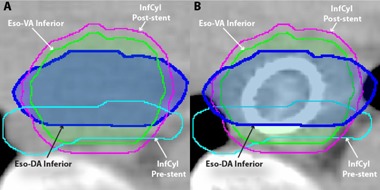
Comparison between VA and DA methods: (a) pre‐stent axial CT; (b) post‐stent axial CT. Note that for more oval‐shaped pre‐stent volumes such as this, the DA method may more closely approximate the pre‐stent esophageal contour.
